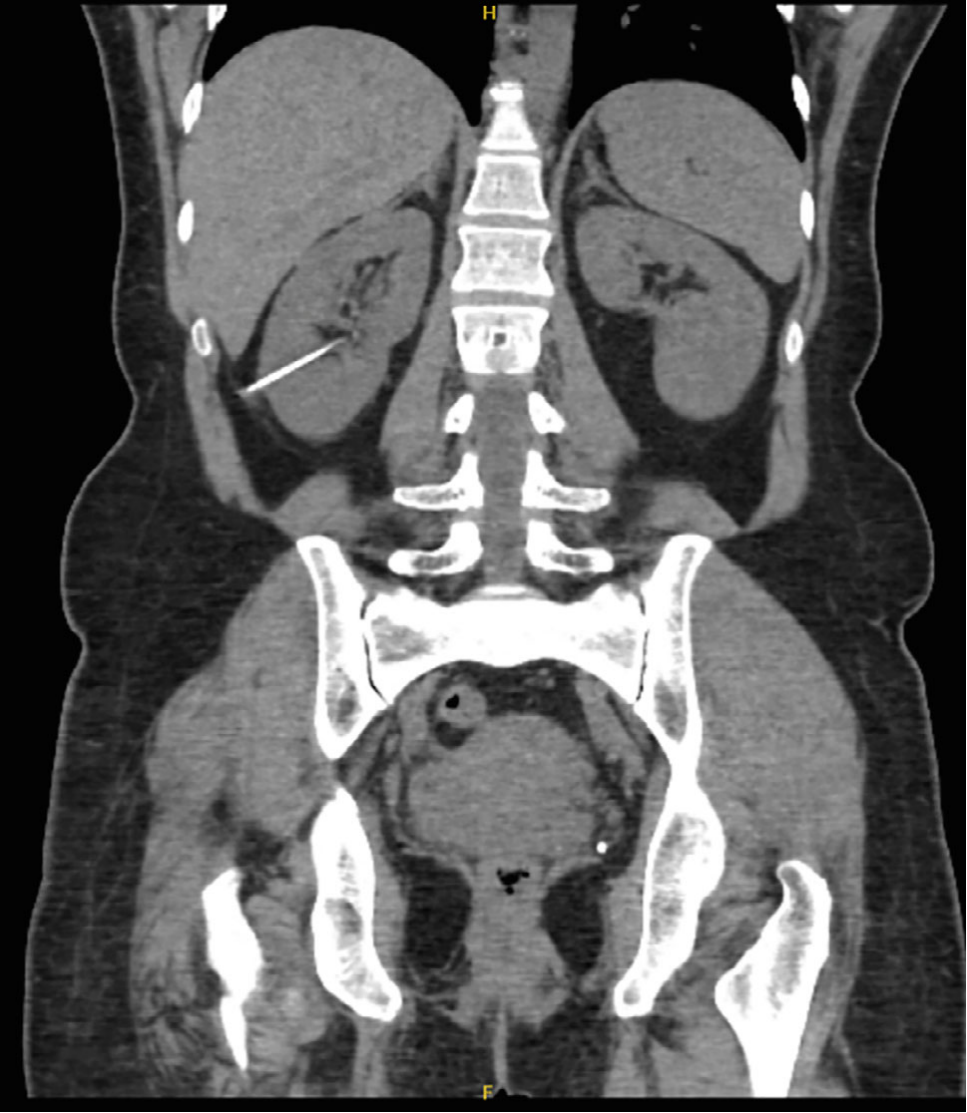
Coronal CT scan with no contrast showing a linear hyperdense foreign body penetrating the posterior cortex of the right kidney and reaching into the lower calyx.
